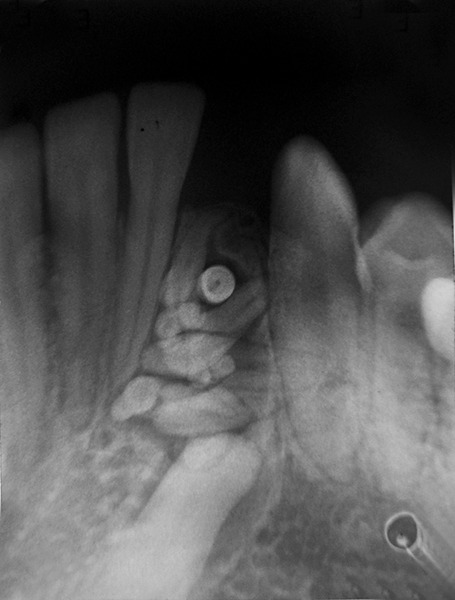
Radiograph of compound odontome
